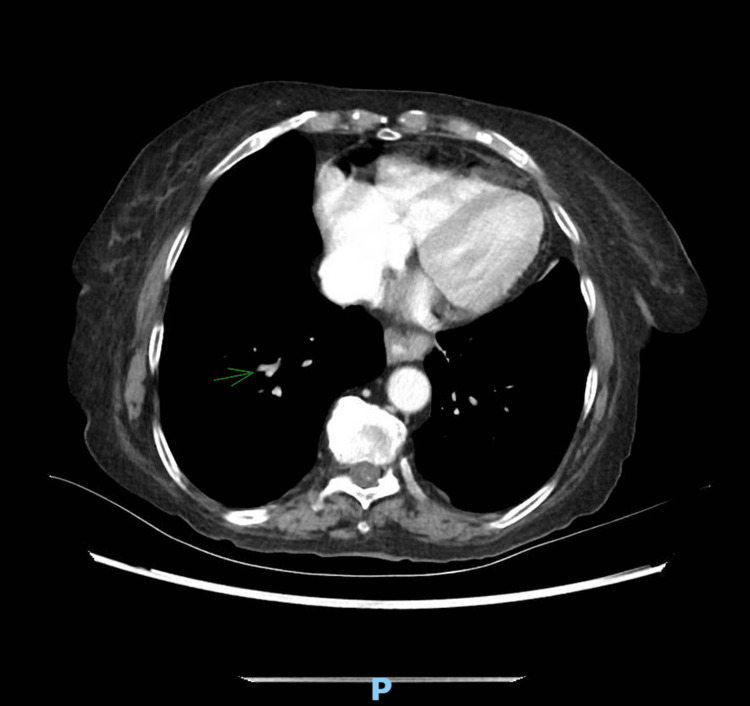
CT abdomen pelvis with incidental hypodensity observed on the right lower lung, suspicious for PE (green arrow) CT, computed tomography; PE, pulmonary embolism.
